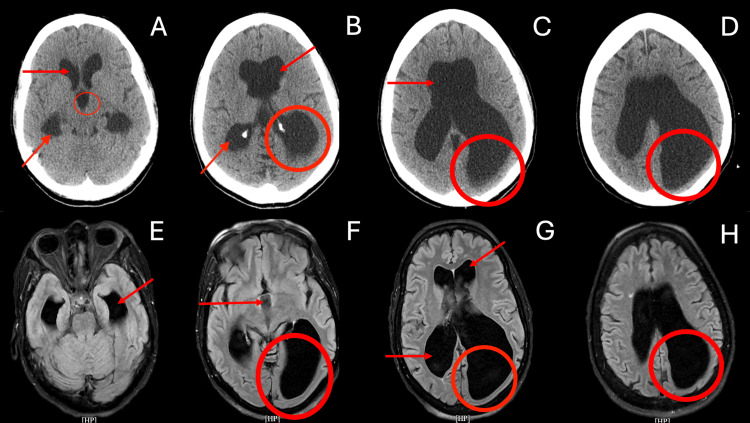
Top row: Head CT scan without contrast. Bottom row: Head MRI T2 with FLAIR. CT overall impression: No acute territorial infarction or acute hemorrhage. An enlarged ventricular system particularly of the lateral ventricles and third ventricle without evidence of dilatation of the fourth ventricle and colpocephaly. A: Arrows show the enlargement of the lateral ventricles at both anterior and posterior locations; the circle shows the enlargement of the third ventricle. B: Arrows demonstrate further enlargement of the lateral ventricles; the circle shows evidence of possible colpocephalic changes. C: The arrow demonstrates severe dilation of the anterior lateral ventricles; the circle shows a clearer *teardrop shape *of the posterior lateral ventricle, showing possible colpocephaly. D: The circle shows continued dilation of the posterior lateral ventricle with colpocephaly. MRI overall impression: No acute infarction, intracranial hemorrhage, or mass lesion. Unchanged dilatation of the ventricular system compared to the previous evaluation. E: The arrow points at enlarged lateral ventricles. F: The arrow shows dilation of the third ventricle; the circle shows severe posterior lateral ventricle dilation. G: Arrows show marked dilation of the lateral ventricles anteriorly and posteriorly; the circle shows evidence of possible colpocephalic changes. H: The circle shows a *teardrop shape*,suggesting possible colpocephaly. CT = computed tomography; MRI = magnetic resonance imaging; FLAIR = fluid-attenuated inversion recovery
